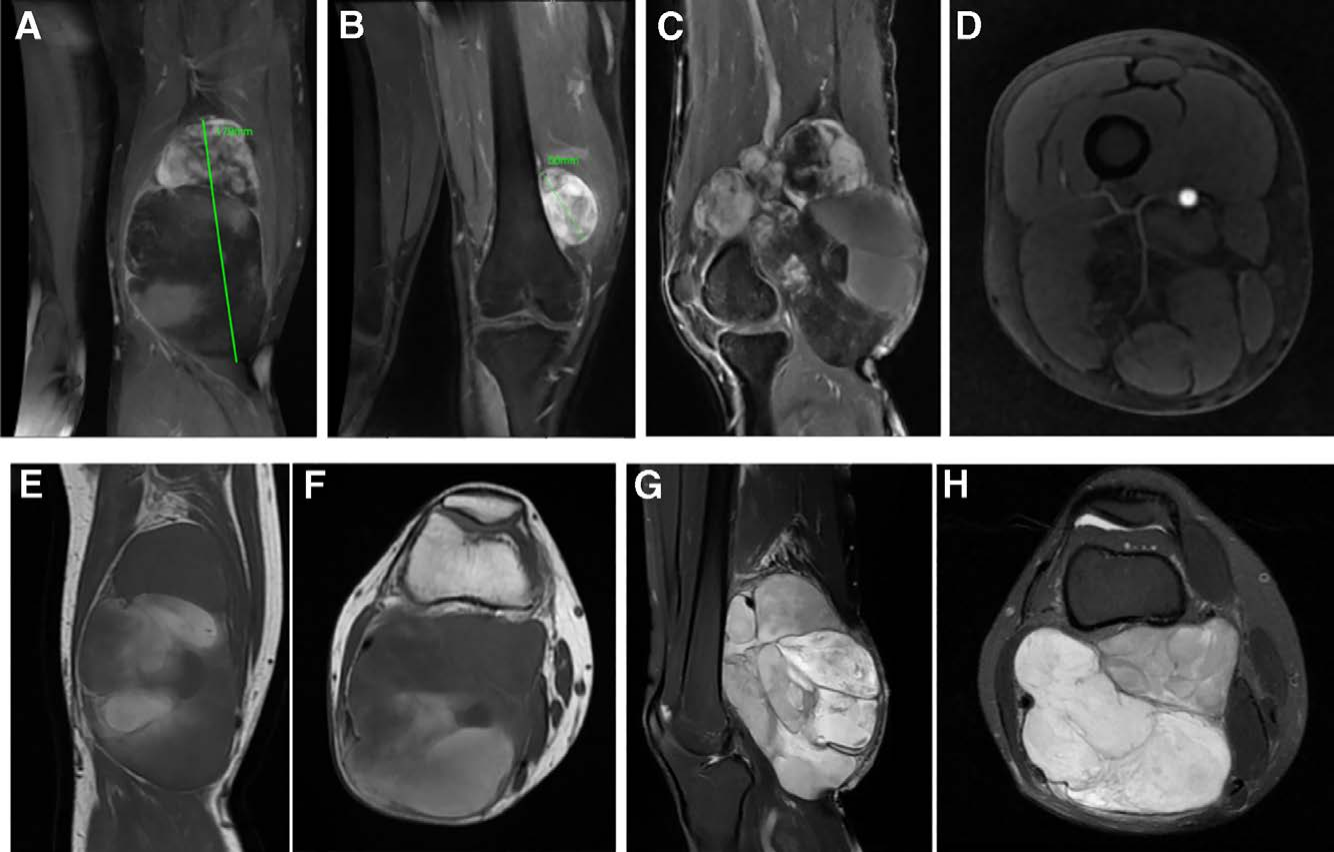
Preoperative MRI images. (A-B) Coronal view of enhanced MRI scan shows a giant mass. (C) Sagittal view of enhanced MRI scan shows that the mass consists of 2 components. (D) Horizontal view of enhanced MRI scan shows the mass is highly vascularized. (E) Coronal view of TIWI of MRI scan shows multilocular cystic changes inside. (F) Horizontal position of TIWI of MRI scan shows high and low mixed signals. (G) Sagittal view of T2WI of MRI scan shows partial non-enhancement of the mass. (H) Horizontal position of T2WI of MRI scan shows The multilocular cystic–solid mass encases the popliteal vessels with septal enhancement.
